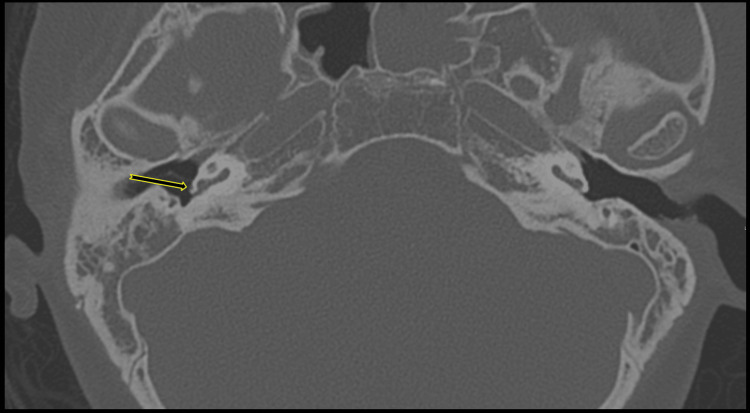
Temporal bone axial CT reconstructions demonstrate the PSA coursing over the right cochlear promontory. A small vascular channel of PSA courses over the lateral surface of the cochlear promontory (long arrow). PSA: persistent stapedial artery
